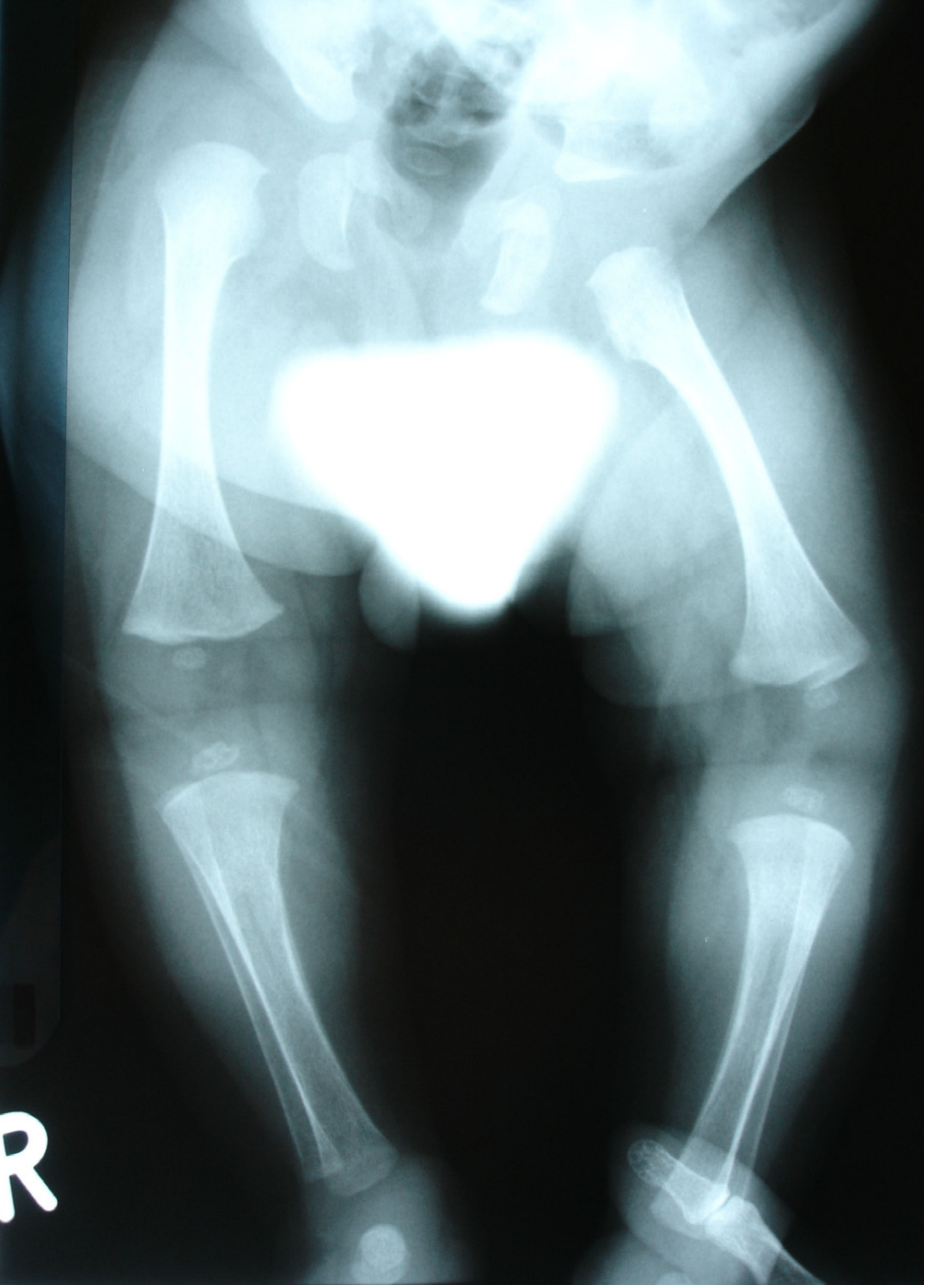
Anteroposterior lower limb radiograph showed epiphyseal dysplasia of the capital femoral epiphyses, hypoplastic ileae, horizontally dysplastic acetabulae, coxa vara, shortening of the femoral necks, broad femora and tibiae with bilateral but asymmetrical degrees of mild bowing. In addition the angulation of the femora was associated with internal thickening of the cortex.
